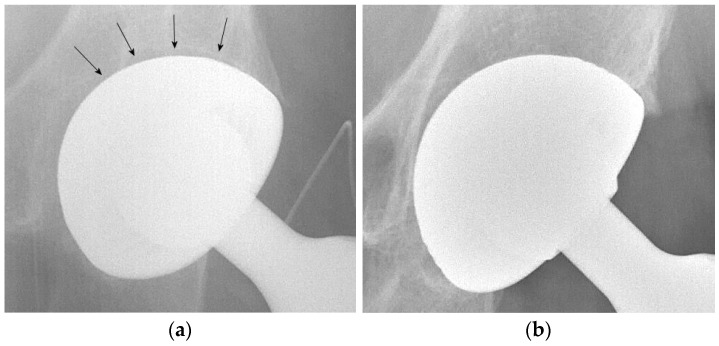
(a) Postoperative radiograph of a DMC showing (arrows) a radiolucent line at the bone-implant interface. (b) Radiographic evidence of implant osteointegration one year after surgery.
